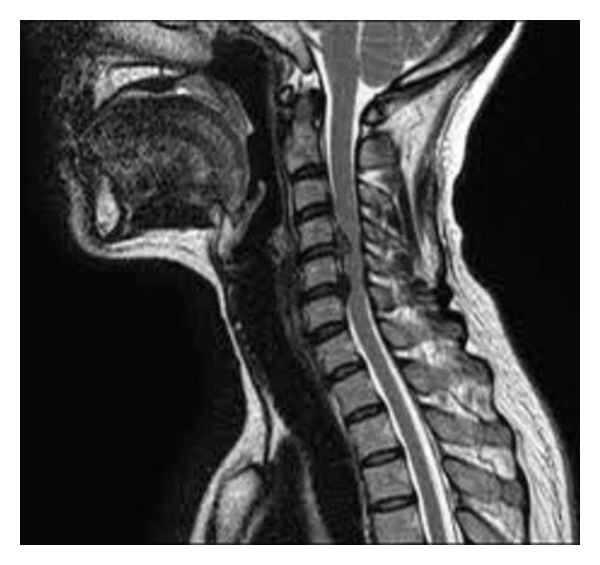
T2-weighted sagittal MRI demonstrating a 2-level CSM with predominantly anterior compression due to soft disc herniation.
